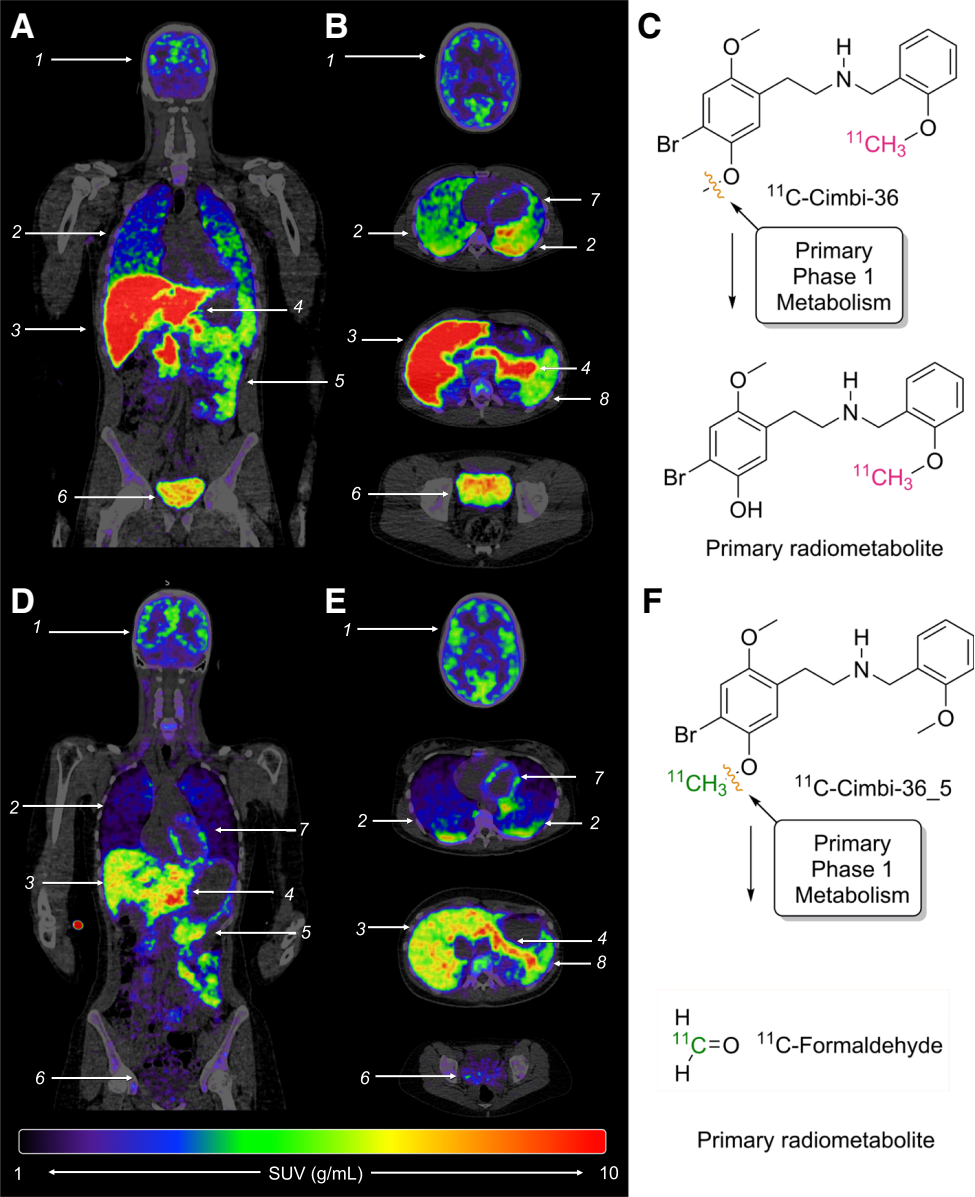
Coronal and horizontal PET/CT fused images of ^11^C-Cimbi-36 (a, b) and ^11^C-Cimbi-36_5 (d, e) 40 min into the scan. Brain (1), lungs (2), liver (3), pancreas (4), small intestines (5), urinary bladder (6), heart wall (7), spleen (8). Primary phase 1 metabolic route resulting in different radiometabolites for ^11^C-Cimbi-36 (c) and ^11^C-Cimbi-36_5 (f)
